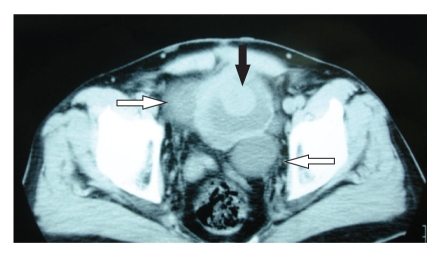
Computed tomography of the lower abdomen showing an exophytic lesion arising from the dome of the bladder (black arrow) and two large diverticulums (white arrows).
